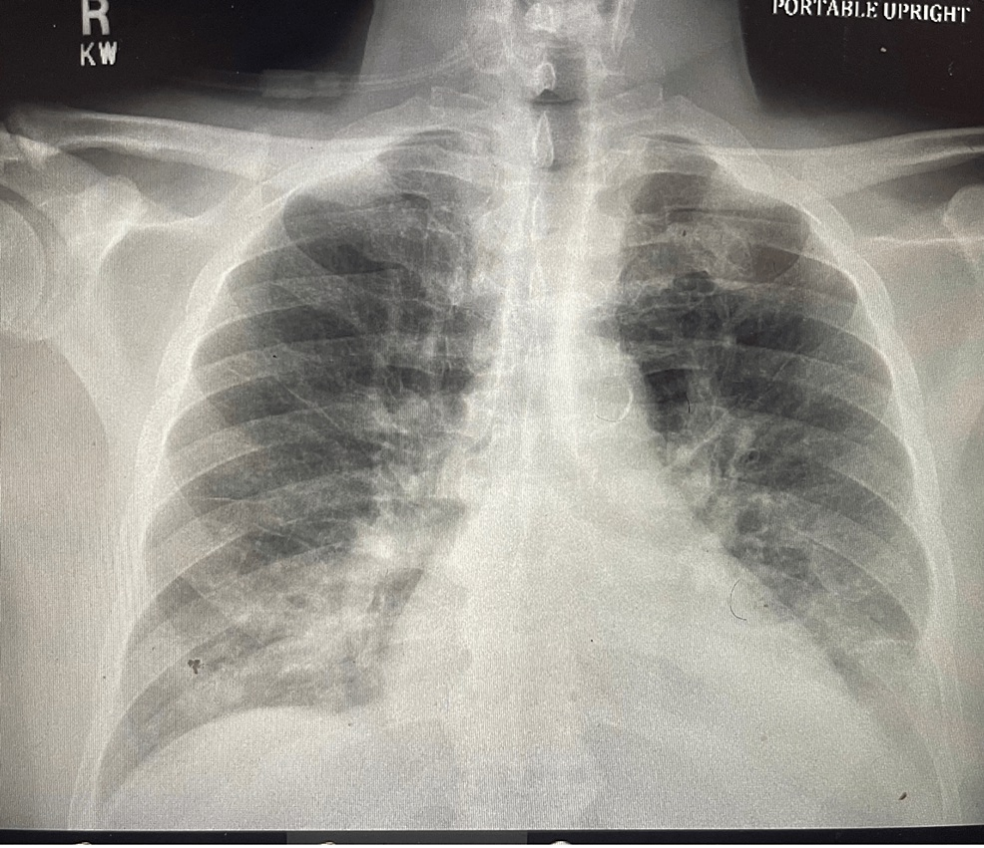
Chest X-ray significant for cardiomegaly with features of congestive heart failure
